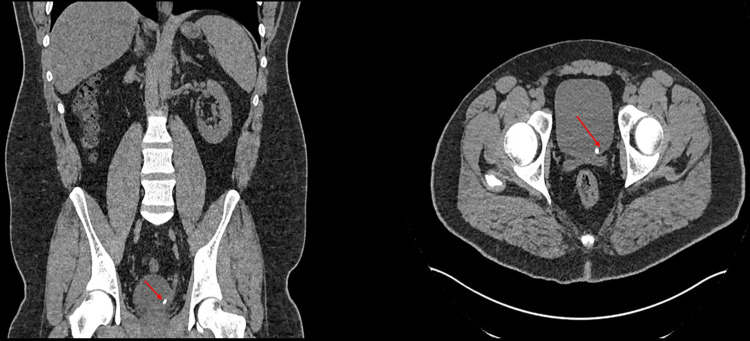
The second CTKUB, taken two months later, shows a persistent left VUJ stone measuring 6 mm. Left: coronal section; Right: axial view. The stone is labelled with red arrows. CTKUB: computed tomography of kidney, ureters and, bladder; VUJ: vesico-ureteric junction
